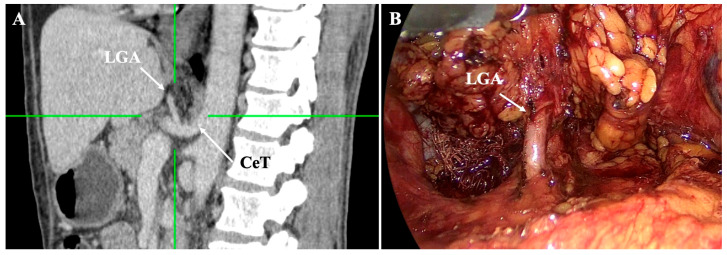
Visualization of typical left gastric artery (LGA) origin from the coeliac trunk (CeT) with computed tomography (A) and intraoperatively (B). Personal archive of Assistant Professor Orestis Lyros.
